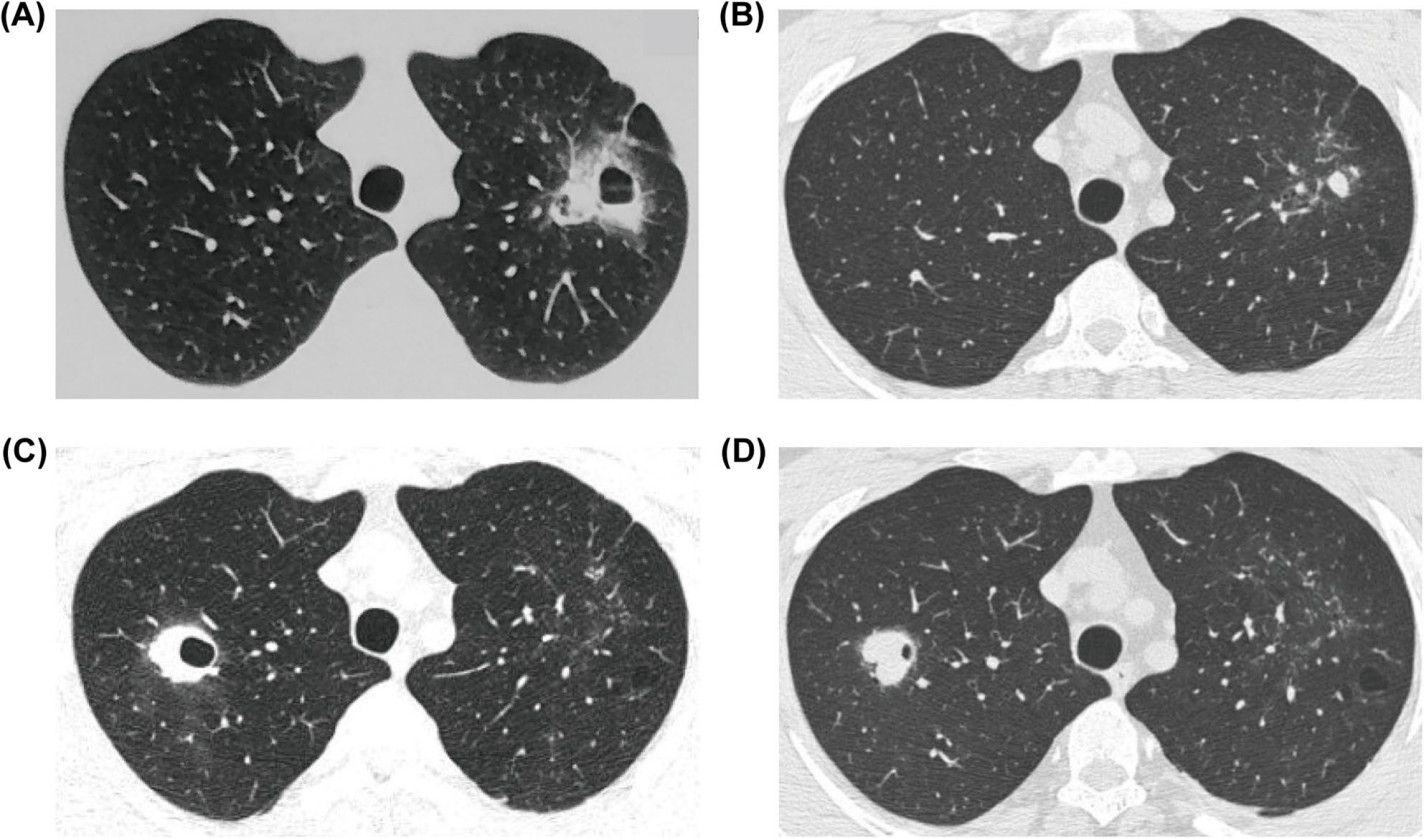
Representative migratory lesions of vascular Ehlers-Danlos syndrome. A 22-year-old man presented with waxing and waning lesions in the lung. (A, B) Chest CT on admission revealed cavity with halo sign in left upper lobe. (C, D) Chest CT taken four months later showed resolution of the prior lesion; however, a new nodule and cavity with a halo sign had developed in the right upper lobe
